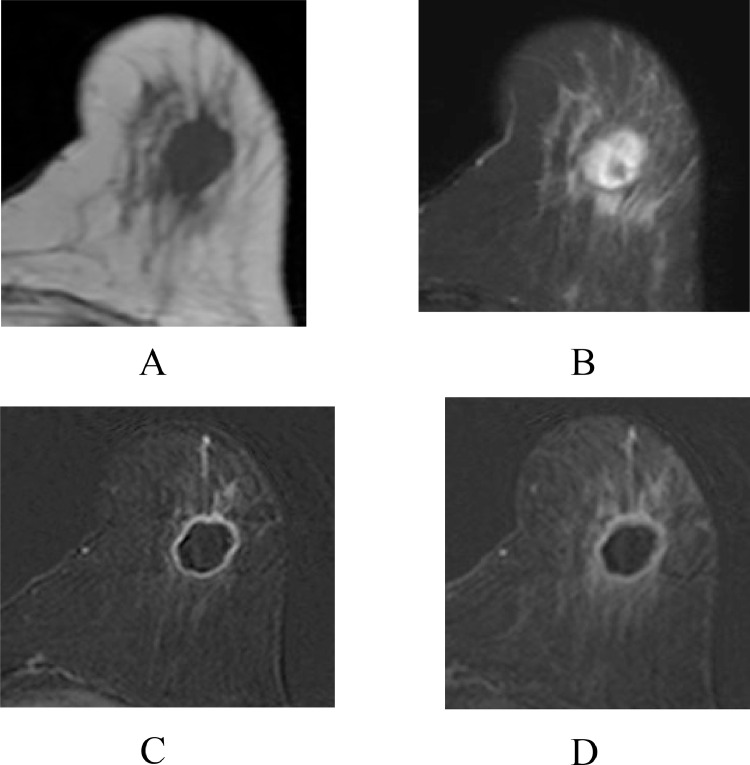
Magnetic resonance imaging (MRI). MRI showed an oval mass with hypo-intensity on T1-weighted images (A), mixed hyper- and hypo-intensity on T2-weighted images (B), and retained rim enhanced until late phase on time-signal intensity curve (C; early phase, D; late phase).
